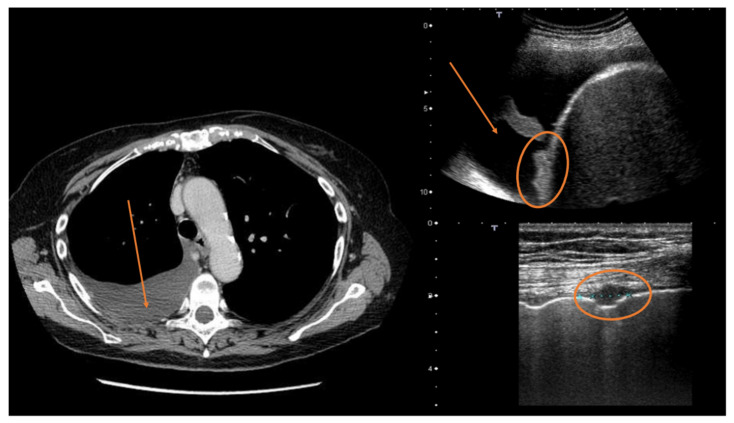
Ultrasound scans of a patient with mesothelioma. On the left, the CT scan shows only pleural effusion (red arrow). The upper right ultrasound scan clearly shows the pleural effusion (red arrow) and diaphragmatic nodulations (red circle). The lower right ultrasound scan shows a parietal pleural nodule (red circle).
